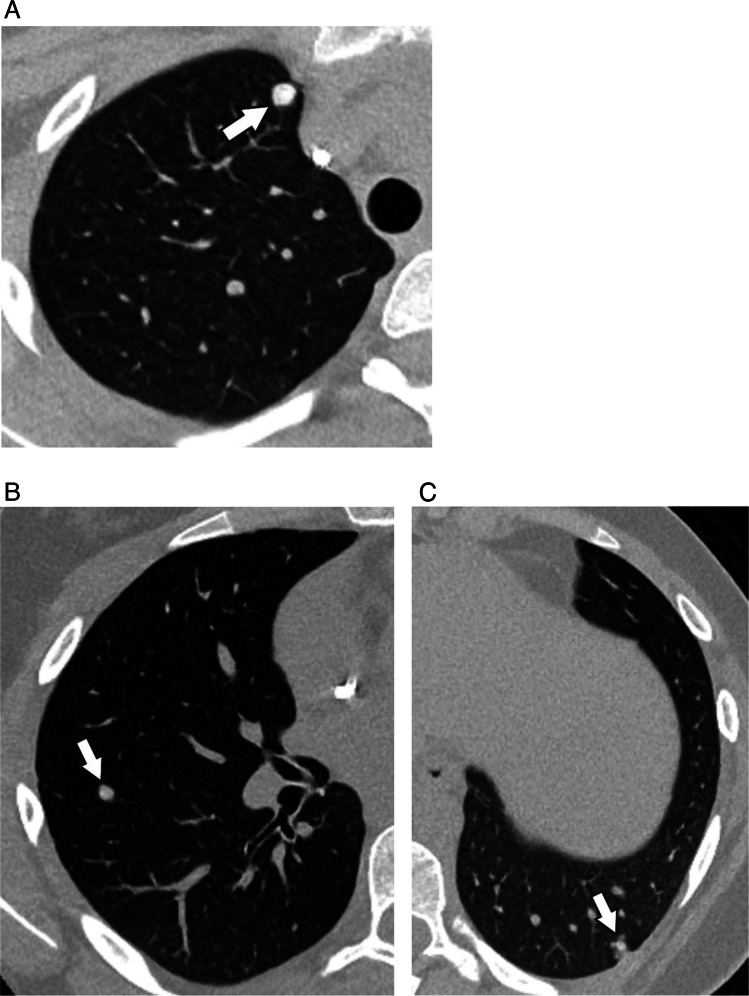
Metastatic osteosarcoma in a 13-year-old female. Non-contrast axial chest CT images (A-C) show multiple bilateral calcified nodules (*arrows*) compatible with metastasis, confirmed with surgical biopsy
